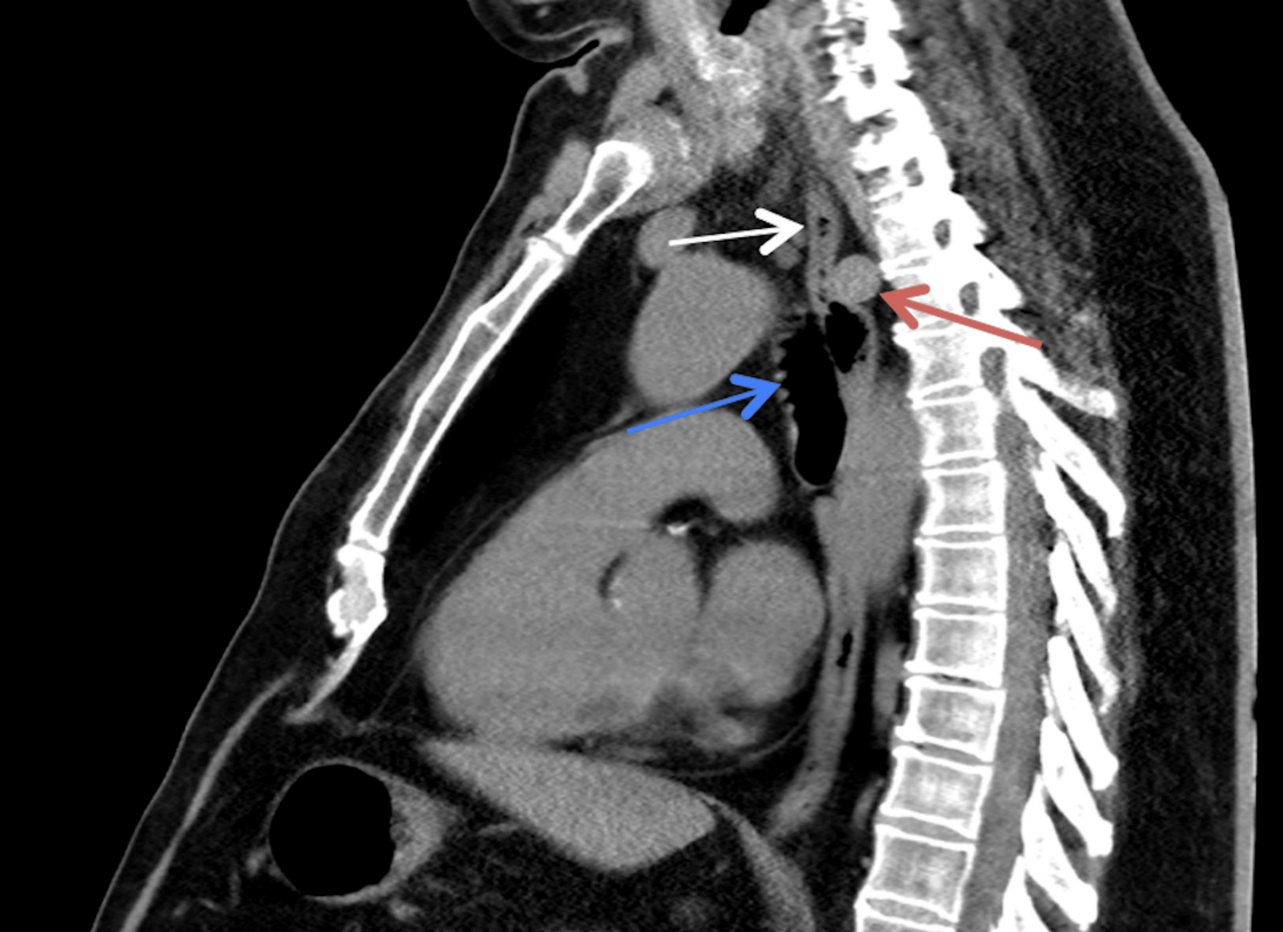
Thoracic CT scan (sagittal view) showing the aberrant subclavian artery (red arrow), thoracic esophagus (white arrow), and trachea (blue arrow) CT: computed tomography
